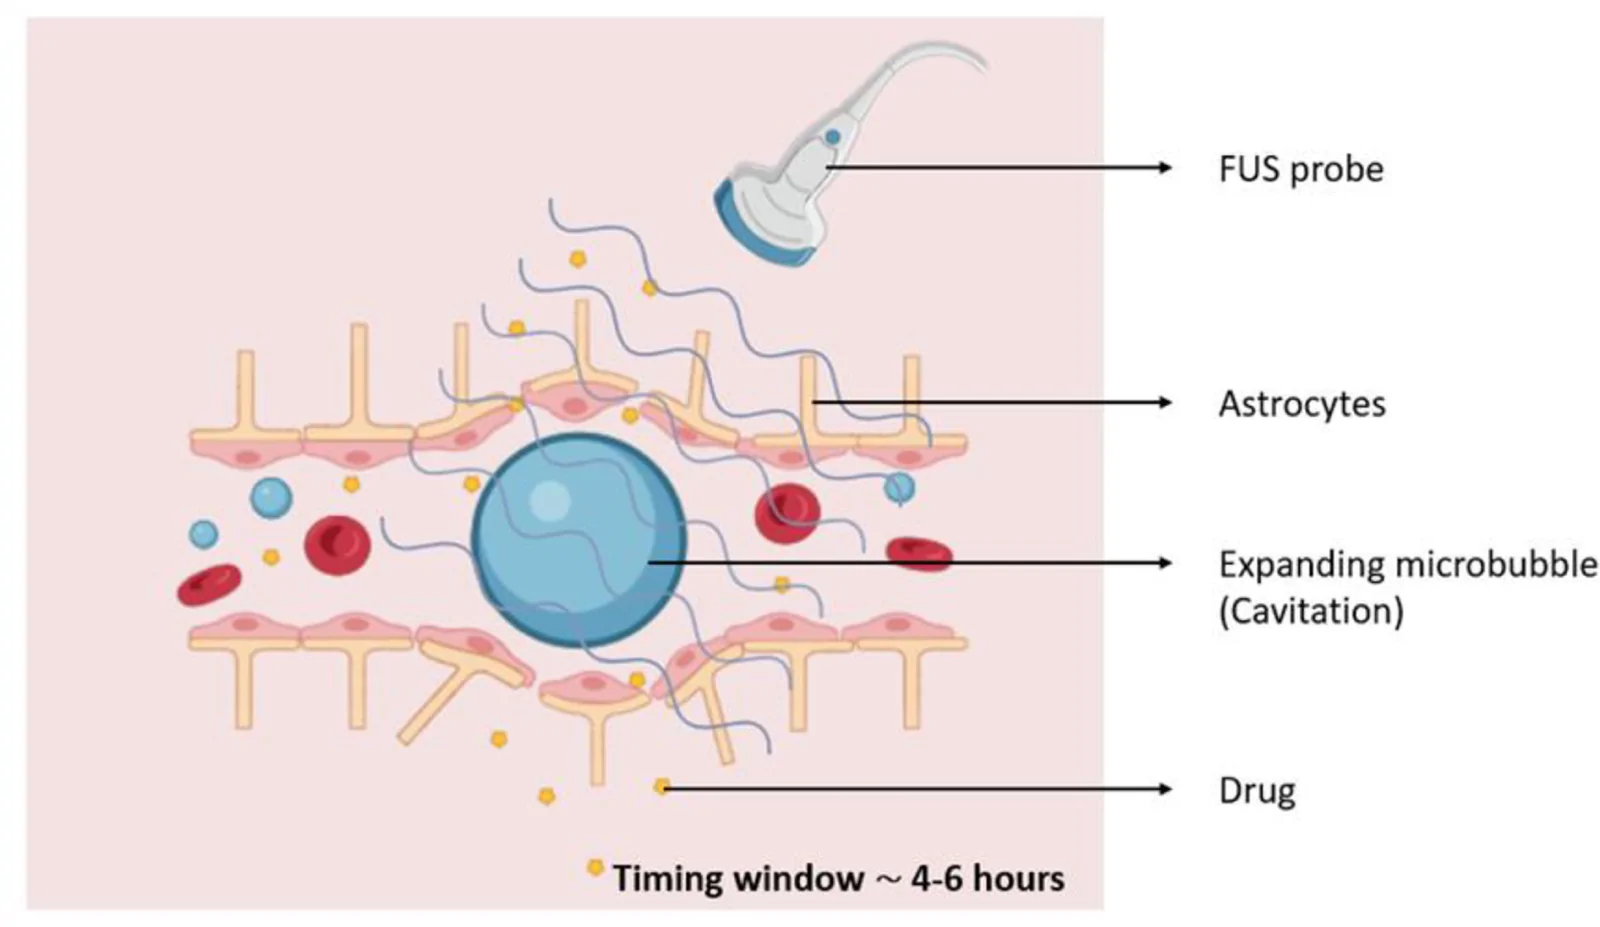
Focused ultrasound-induced disruption of the BBB and the BCSFB for enhanced drug delivery in CNS-TB.
